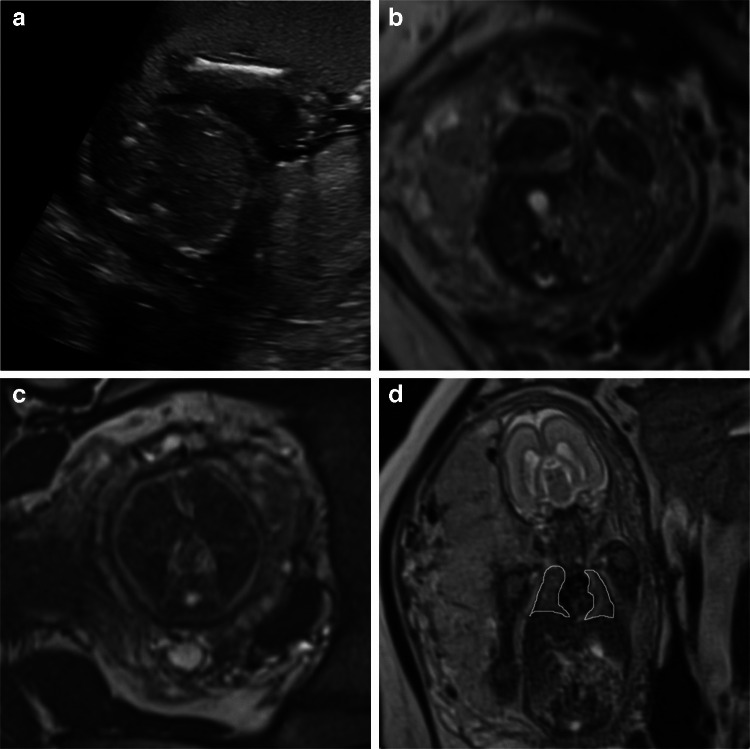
a Axial ultrasound of a 20-week gestation male fetus showing anhydramnios and absent bilateral kidneys. b MRI imaging obtained the same day confirming absent kidneys, bilaterally, on the axial Single-Shot Fast Spin Echo (SSFSE) image. Note the absence of amniotic fluid. c Axial SSFSE image at the level of the pelvis confirming non-visualized urinary bladder. d Coronal SSFSE image demonstrating Potter’s Ssequence with compression of the fetus and T2-hypointense hypoplastic lungs (white outline)
